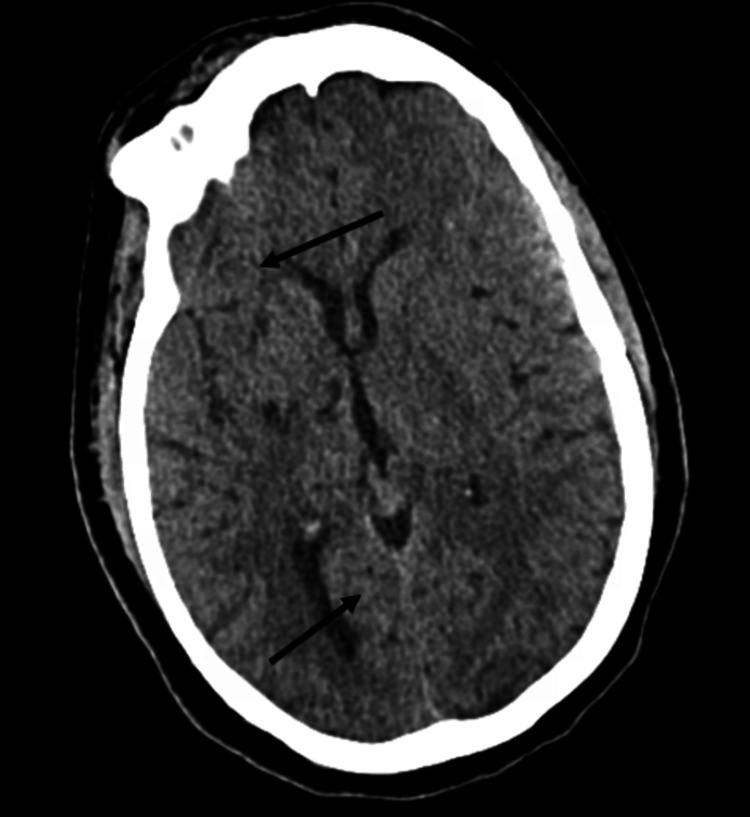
Axial non-contrast CT of the brain The image indicated right frontal vasogenic edema, consistent with opportunistic CNS infection and determined to be evidence of new brain lesions, rather than an acute ischemic infarct. The image also showed development of edema within the right cerebellum with mild mass effect on the fourth ventricle, suggestive of an underlying lesion (lower arrow) in the setting of vasogenic edema in the posterior right frontal lobe in the location of the previously known enhancing lesion (upper arrow), consistent with recurrent toxoplasmosis CT: computed tomography; CNS: central nervous system
